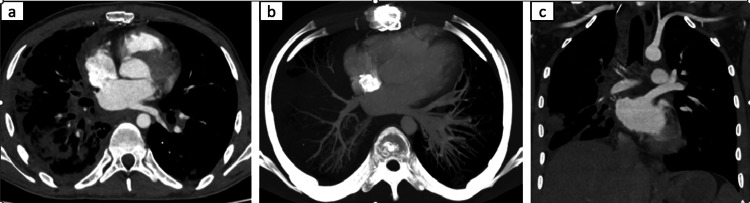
(a) Axial CT. (b) Axial CT, MIP. (c) Coronal CT On follow-up contrast-enhanced CT postoperatively, (a,c) the reconstructed left atrium appeared normal, with no evidence of residual tumor, and (b) the reimplanted right pulmonary vein CT: computed tomography; MIP: maximum intensity projection
